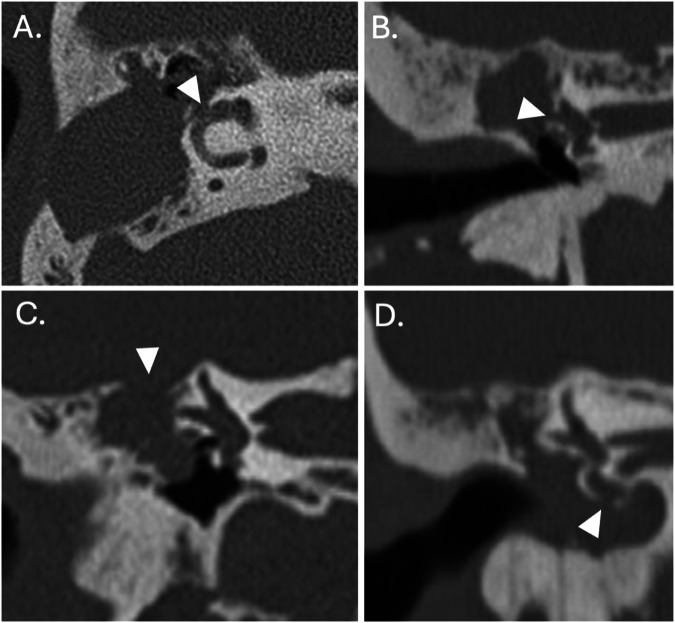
Cholesteatoma-mediated bone erosion on CT. Axial (A) and coronal (B) CT sections through the right temporal bone of a patient with an extensive tympanomastoid cholesteatoma demonstrating erosion of the lateral semicircular canal (arrowheads). Coronal CT reconstruction (C) in another patient with an extensive right epitympanic cholesteatoma demonstrating tegmental erosion (arrowhead). A coronal reconstruction (D) in a different patient with an extensive middle ear cholesteatoma demonstrates erosion of the basal turn of the cochlea (arrowhead)
